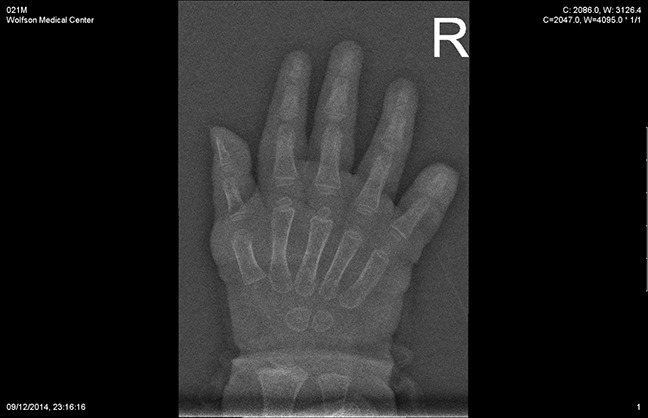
Radiograph of the distal phalanx amputation, AP view
